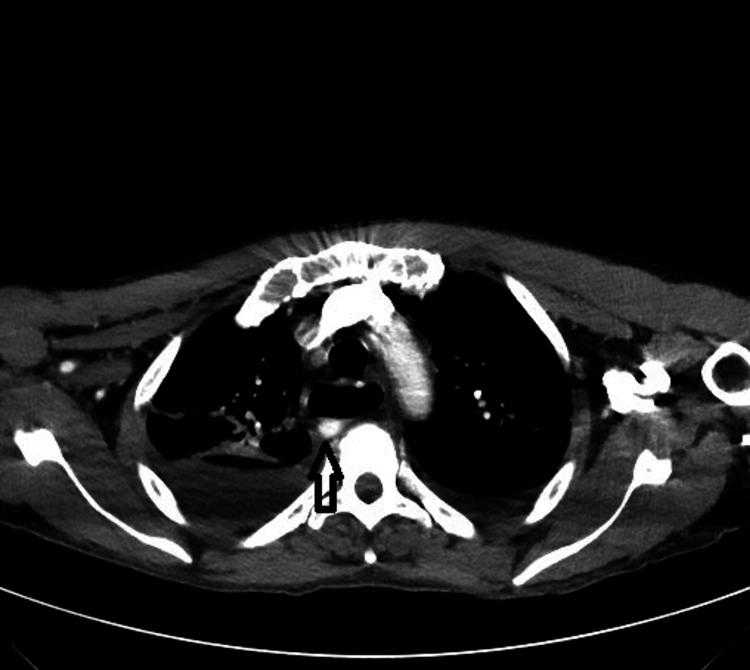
Chest CT angiography Chest CT angiography showing aberrant right subclavian artery (arrow)
